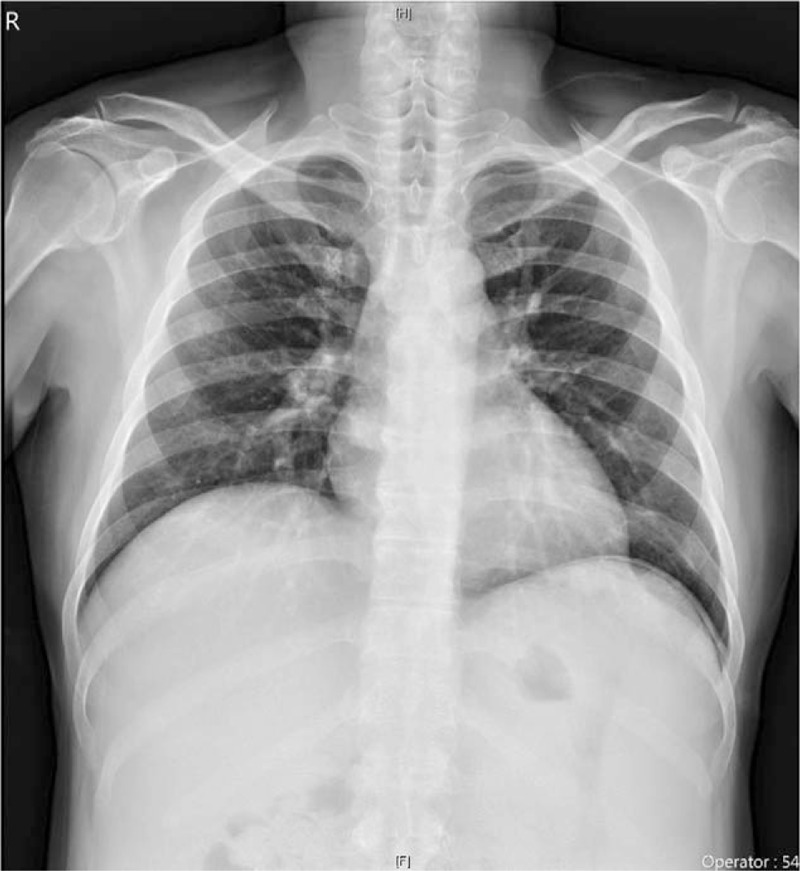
Chest radiography. Chest X-ray demonstrating the presence of free air below left side hemidiaphragm.
